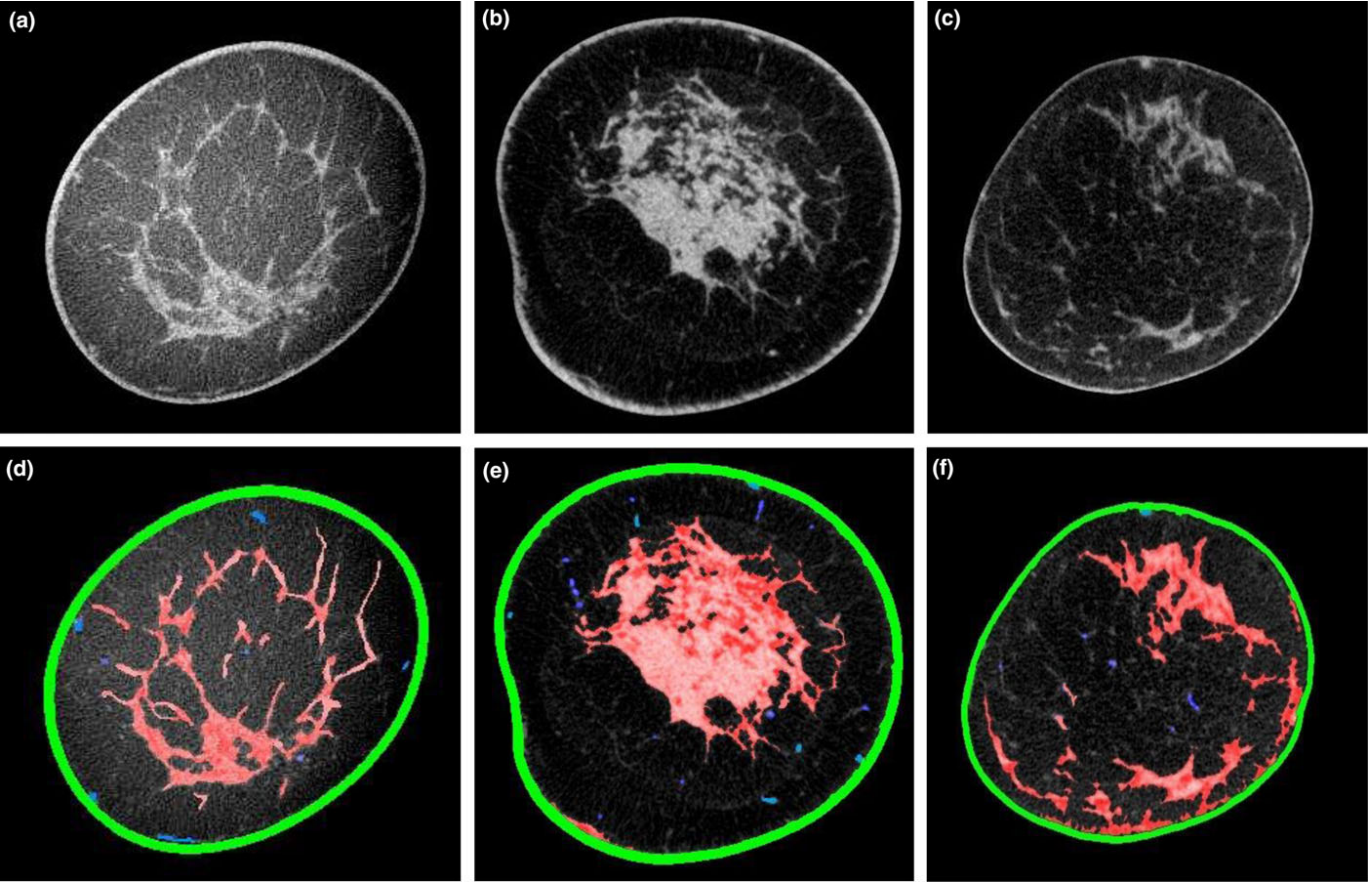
Three examples of (a, b, and c) original (coronal) slices from different breast CT patient images, and (d, e, and f) respective results of the automatic tissue classification, showing the skin, the fibroglandular tissue, the vasculature, and the adipose tissue. [Color figure can be viewed at http://wileyonlinelibrary.com]
